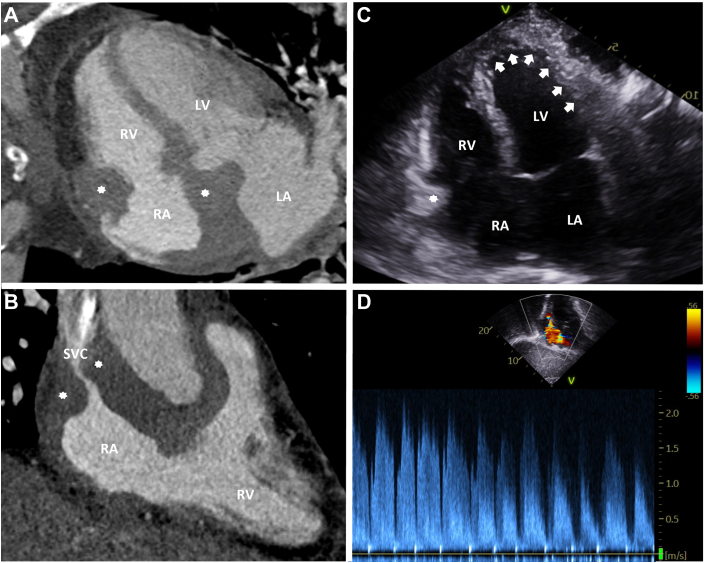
Multimodality Imaging of a Right Atrial Mass With SVC Obstruction (A) Four-chamber multiplanar reconstruction from a cardiac computed tomography angiogram showing a large intracardiac mass (asterisks) involving the RA. (B) Right ventricular inflow-outflow view demonstrating the same mass (asterisks) with SVC stenosis. (C) Transthoracic echocardiogram in the apical 4-chamber view showing the intracardiac mass (asterisk) and a complex pericardial effusion (arrows). (D) Continuous-wave Doppler from the suprasternal view revealing elevated flow velocities in the SVC, consistent with hemodynamically significant SVC obstruction. LA = left atrium; LV = left ventricle; RA = right atrium; RV = right ventricle; SVC = superior vena cava.
